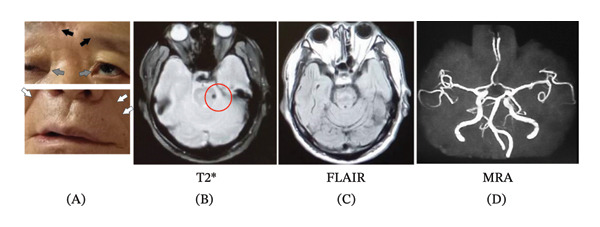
Facial view (A) and initial MRI image. (A) Facial view showing facial asymmetry. The left forehead lacks wrinkling (black arrow), the palpebral fissure is wider on the left than on the right (gray arrow), and the left nasolabial fold is flattened (white arrow). On MRI, a dot‐like hypointensity is observed in the left pons on T2^∗^‐weighted imaging ((B) red circle), and the same region shows a focal hypointense spot on FLAIR imaging (C). No vascular malformations are detected on MRA (D).
